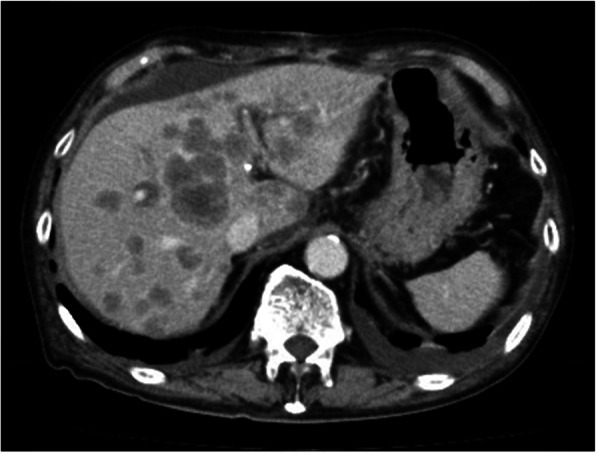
Multiple liver metastases and ascites on the liver surface were observed by CT (axial section image) 1 month after surgery
